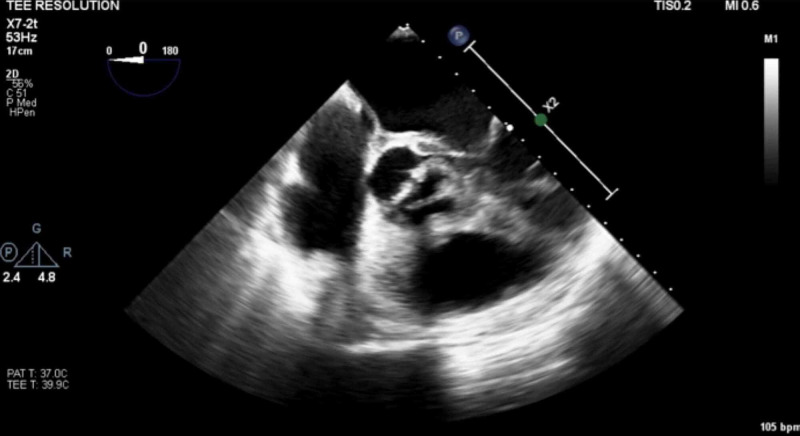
Transesophageal echocardiogram showing bicuspid aortic valve (BAV)
